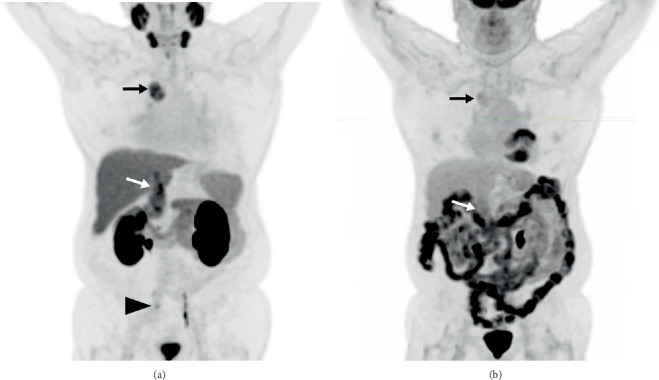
Maximum intensity projections (MIPs) of the baseline ^18^F-DCFPyL PET/CT (a) and FDG PET/CT (b) studies. In addition to the pelvic nodal metastasis from prostate cancer noted on PSMA PET (a, arrowhead), additional sites of abnormal uptake ultimately led to a new diagnosis of ccRCC. PSMA PET/CT demonstrated greater radiotracer uptake than FDG PET/CT at both the right paratracheal metastasis (a and b, *black arrows*) and tumor thrombus (a and b, *white arrows*).
